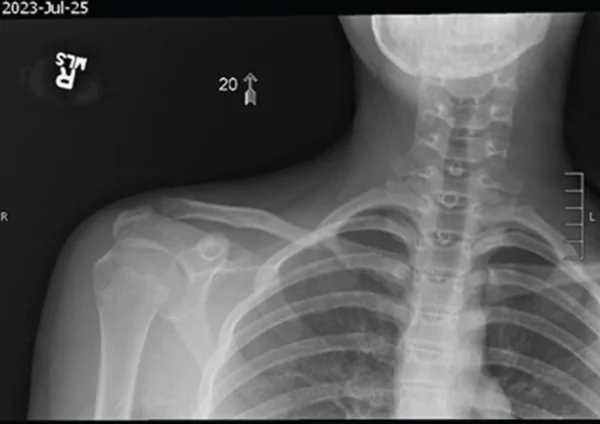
Day 15 radiographs demonstrating radiographic findings consistent with early callus formation and interval healing.
